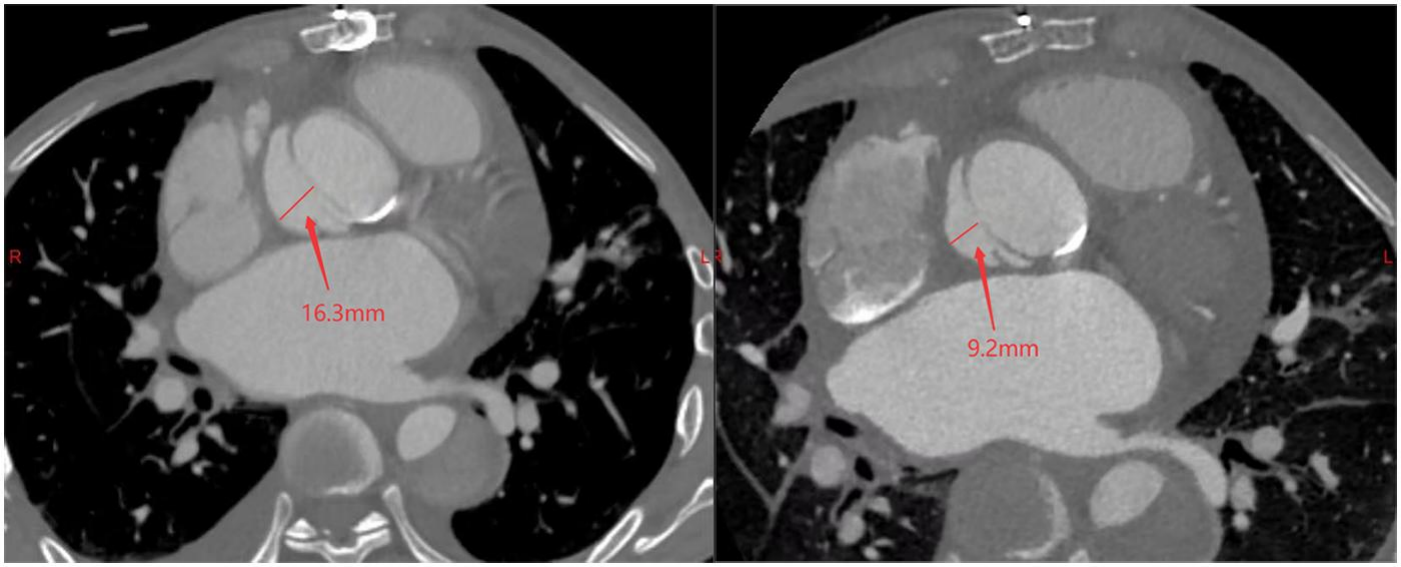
Pre-procedural (left) and post-procedural (right) CT scans demonstrating the pseudoaneurysm at its maximum width.
